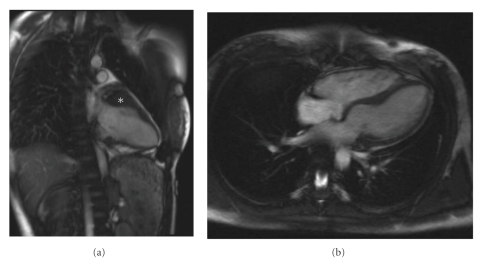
Focal hypertrophy noted on the proband's cardiac MRI. (a) 2-chamber end-diastole view. *marks the focal thickening (2.7 cm, thick × 1.8 cm, length) of the anterior wall at the base of the left ventricle. (b) 4-chamber end-diastole view.
